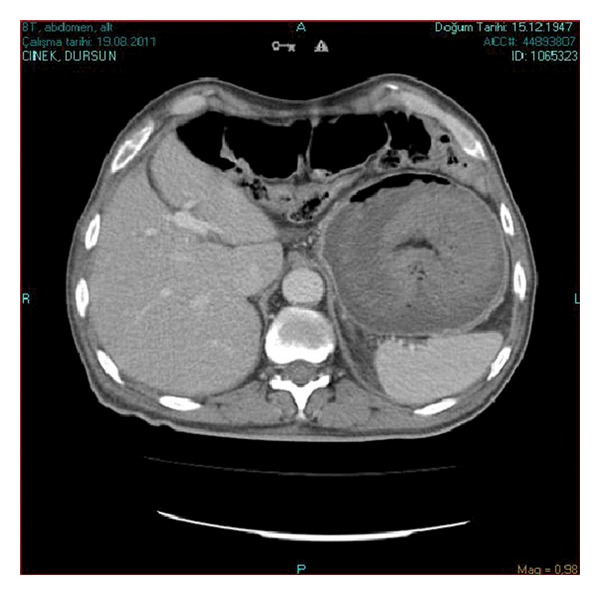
Emergency CT scan of the abdomen. Another section showing a dilated stomach with intragastric nonhomogeneous mass compatible with bowel loops.
